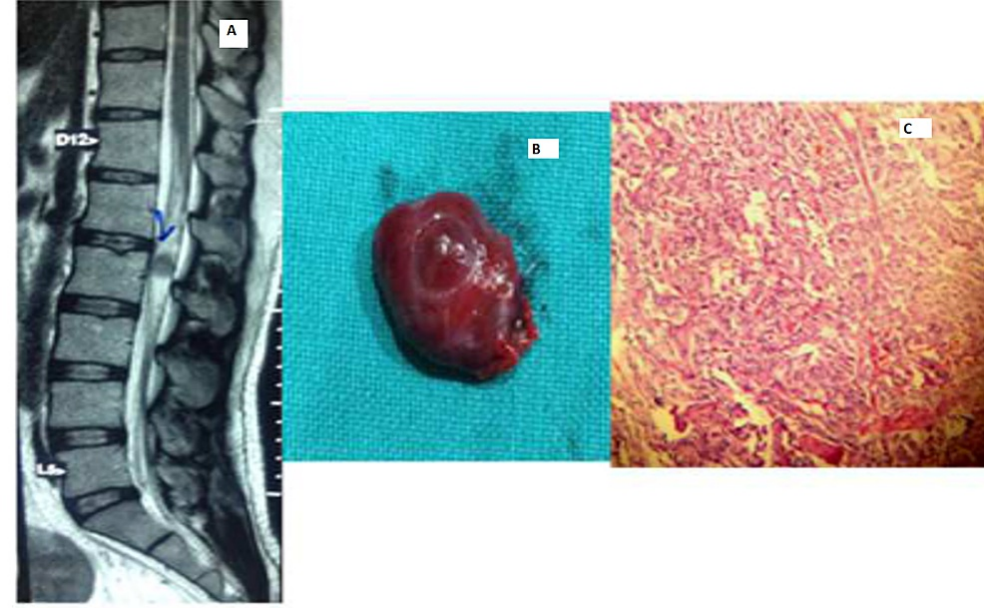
Paraganglioma of cauda equina region A: MRI T2-weighted sagittal image with hypo intense lesion. B: Cauda equina paraganglioma sample after excision. C: Strong synaptophysin expression in tumor cells.
